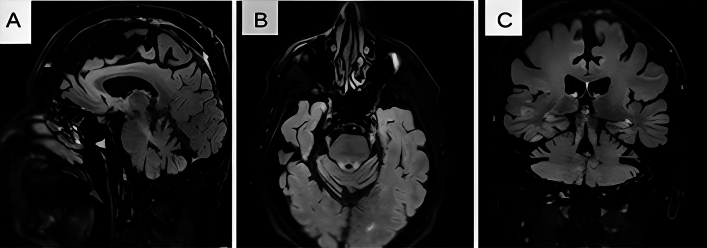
T2 FLAIR magnetic resonance imaging of the brain A sagittal view, B axial, and C coronal views, showing high signal intensity in the superior cerebellar peduncle and atrophy of the superior cerebellar vermis
